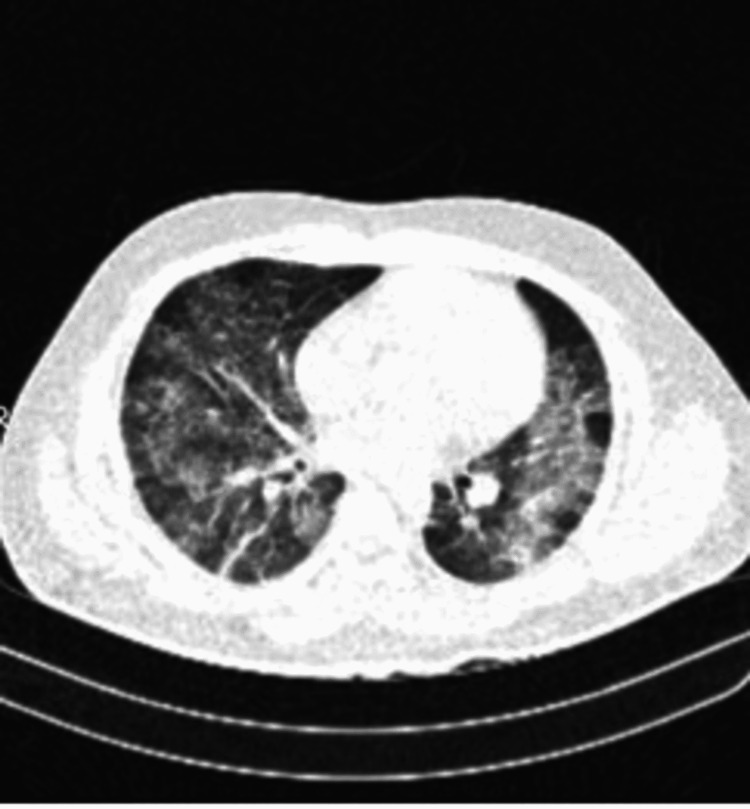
HRCT of lungs showing multiple ill-defined patchy ground-glass opacities with septal thickening and patchy areas of consolidation in bilateral lung fields HRCT - high-definition computed tomography
